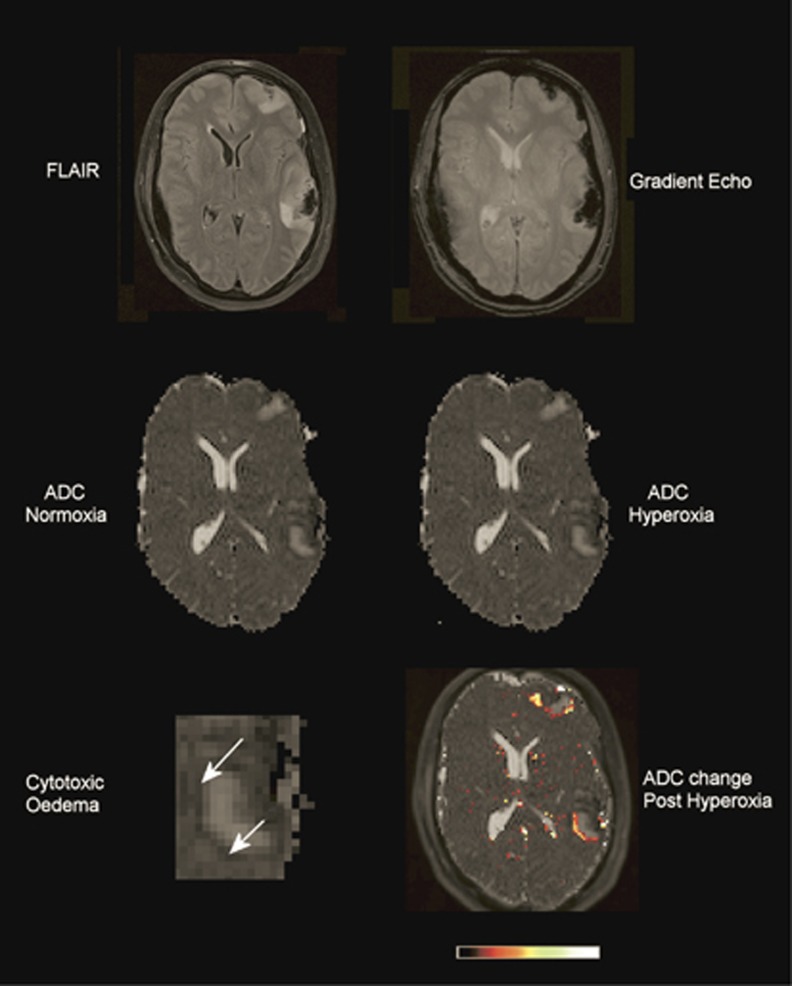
Traumatic penumbra. Fluid-attenuated inversion recovery (FLAIR), gradient echo, and apparent diffusion coefficient (ADC) images at normoxia and hyperoxia demonstrating contusions within the left frontal and temporal parietal regions. These lesions have a hemorrhagic core shown by low signal on the gradient echo corresponding to the presence of blood degradation products, surrounded by a region of ‘vasogenic edema' with high signal on FLAIR and ADC. Around these lesions is a hypointense rim consistent with ‘cytotoxic edema', an example of which is shown at higher magnification and identified by the arrows. The final image has a color map showing the ADC increase calculated from the difference between the ADC images after hyperoxia. This highlights that the increase in ADC occurs predominantly within this border zone immediately surrounding the contusions.
